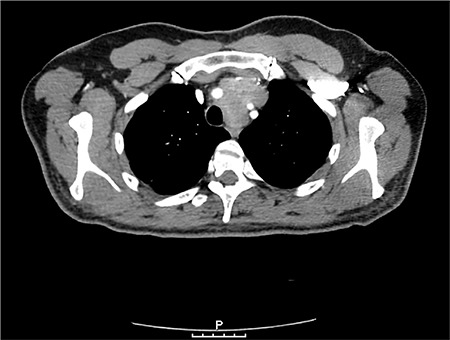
Axial CT slice of a solitary fibrous tumor located in anterior mediastinum.
